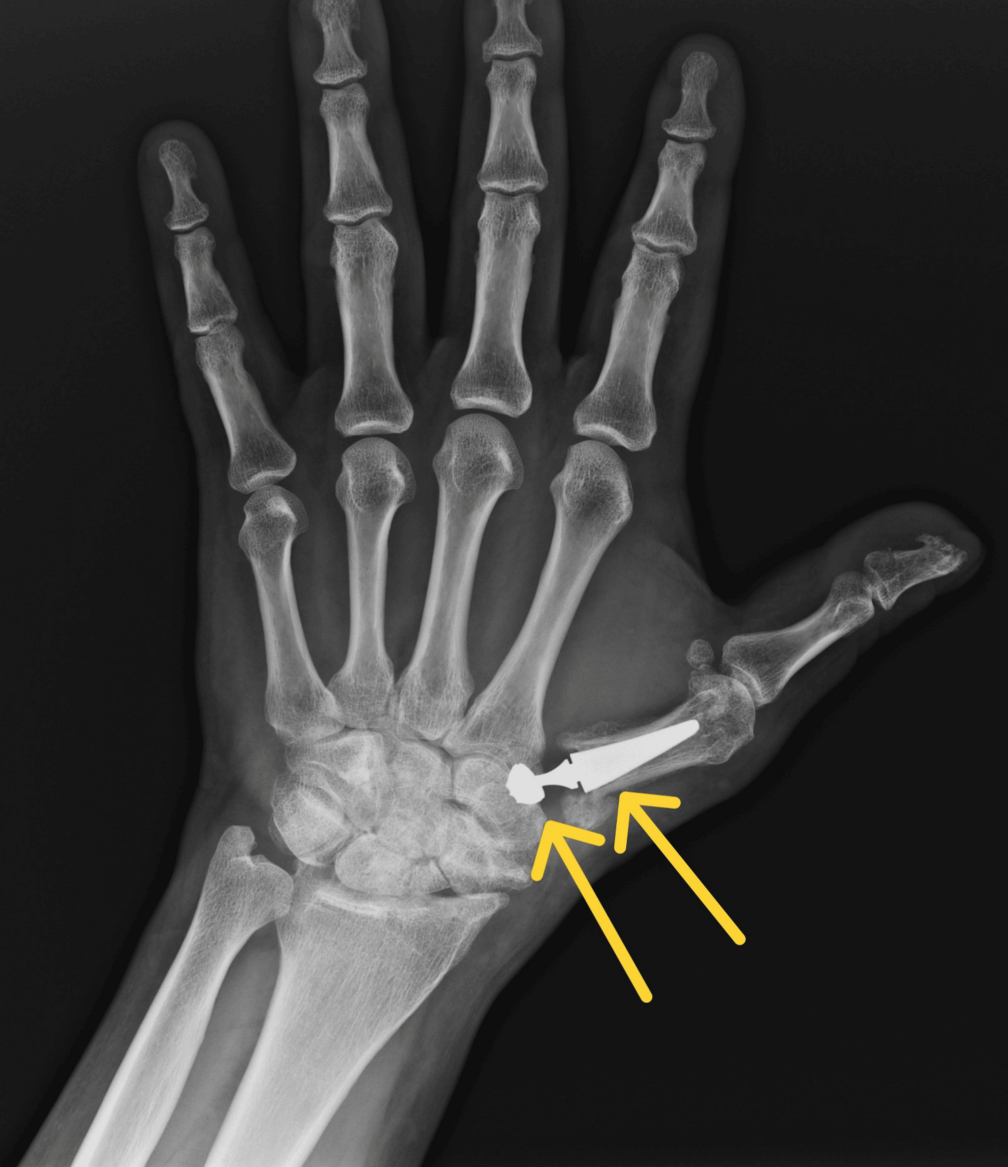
X-ray in A-P projection obtained two years after surgery A-P: anterior-posterior
